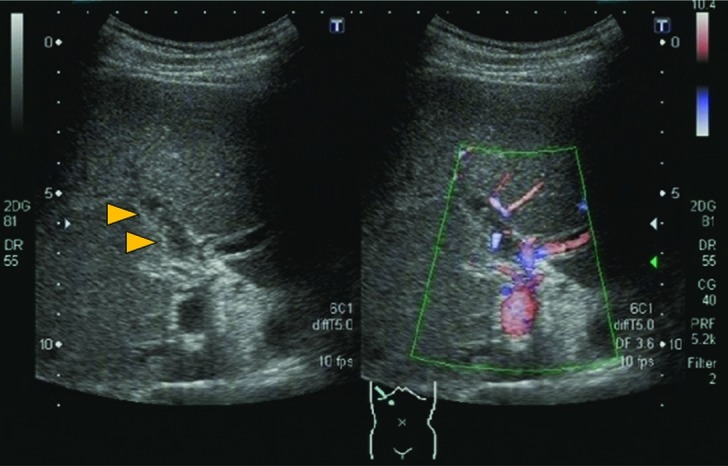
Abdominal ultrasonograpy (US) findings on admission. Left: B-mode US revealed a solid lesion with a mixed hyper- and hypoechoic pattern (arrowheads) in the right branch of the hepatic portal vein. Right: color Doppler US showed neither blood flow through the right branch nor the signs of portal vein dilatation.
